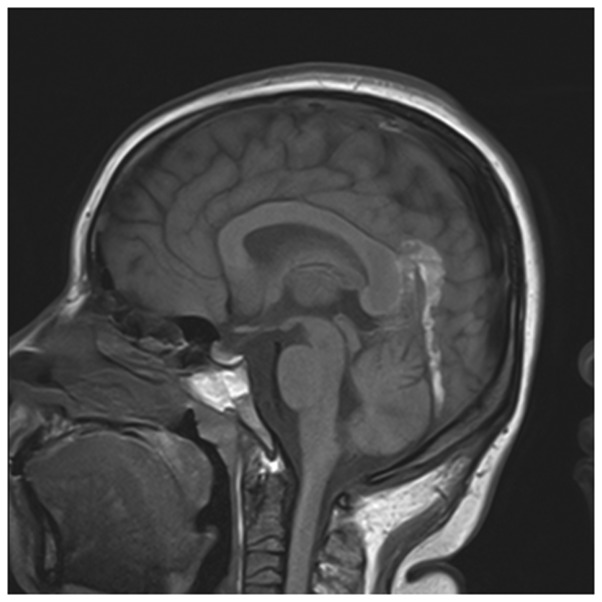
Magnetic resonance imaging (MRI) T1-weighted image displayed mixed signals for the straight sinus and vein of Galen and corpus callosum splenium swelling.
